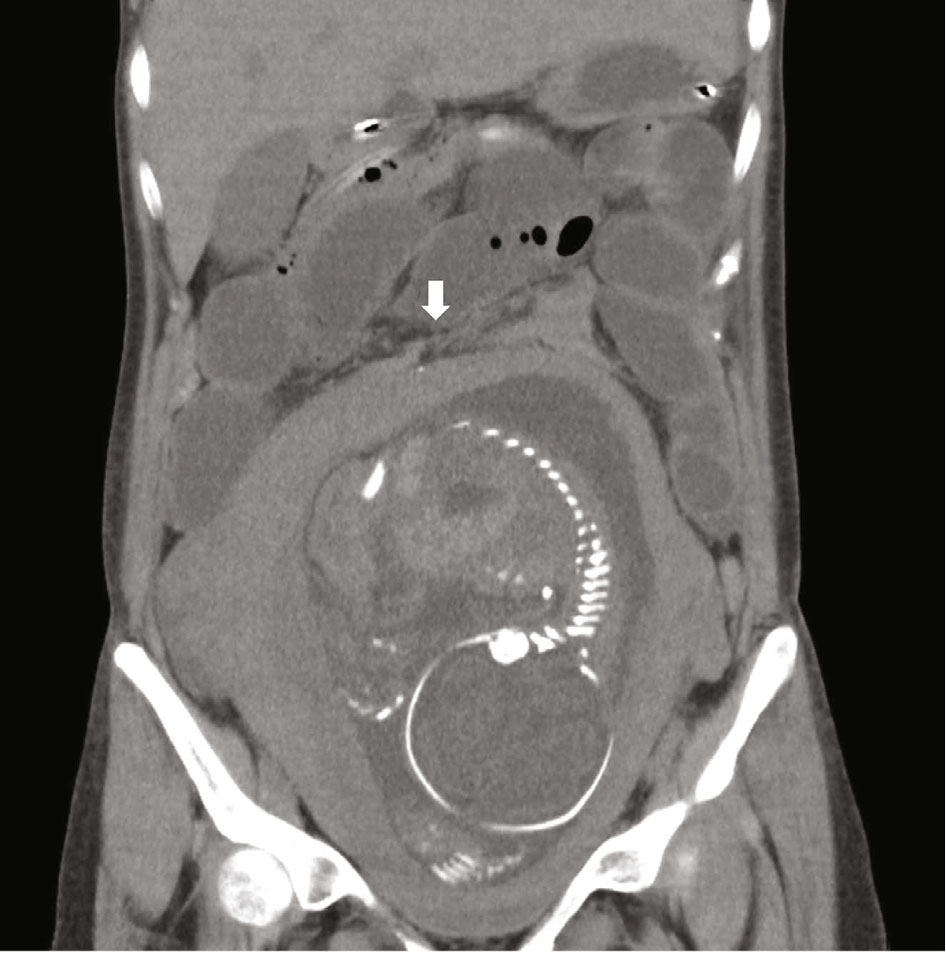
CT findings of adhesive small intestine at 26-week gestation. An arrow indicates adhesion site.
